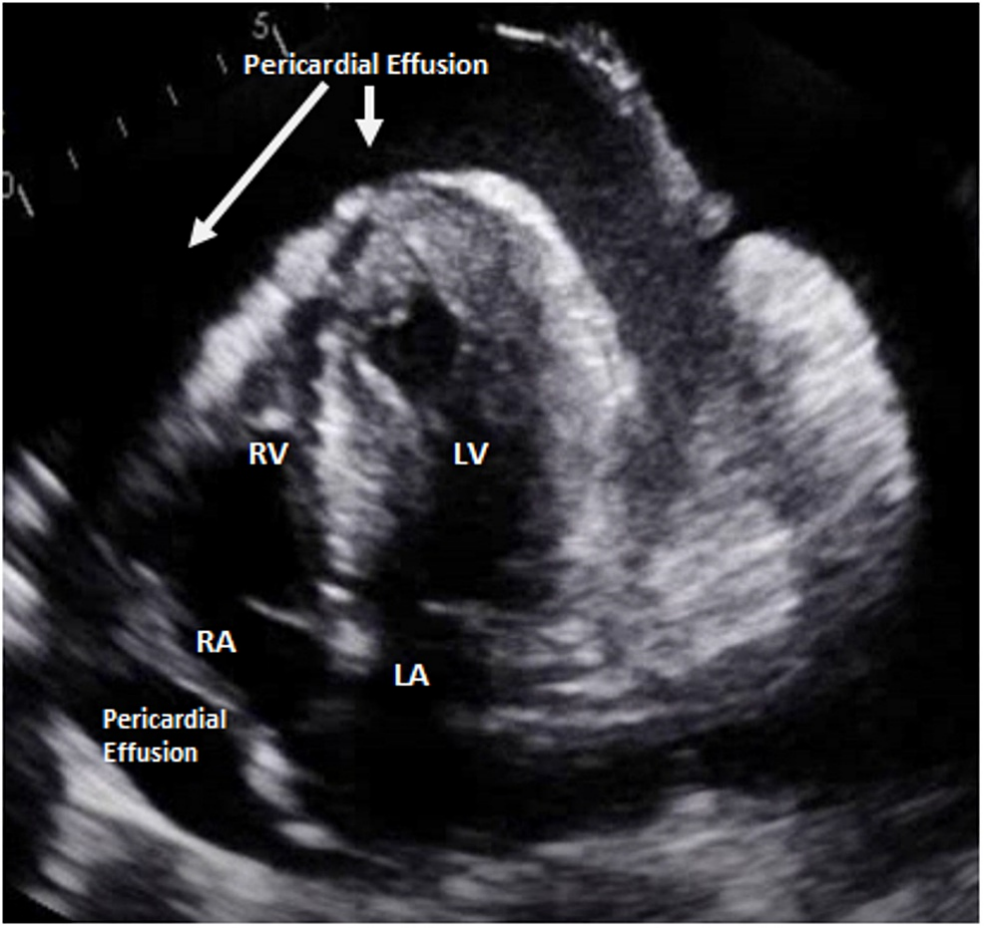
Four-chamber echocardiography demonstrating large pericardial effusion and right chamber collapse. RV, right ventricle; LV, left ventricle; RA, right atrium; LA, left atrium.
